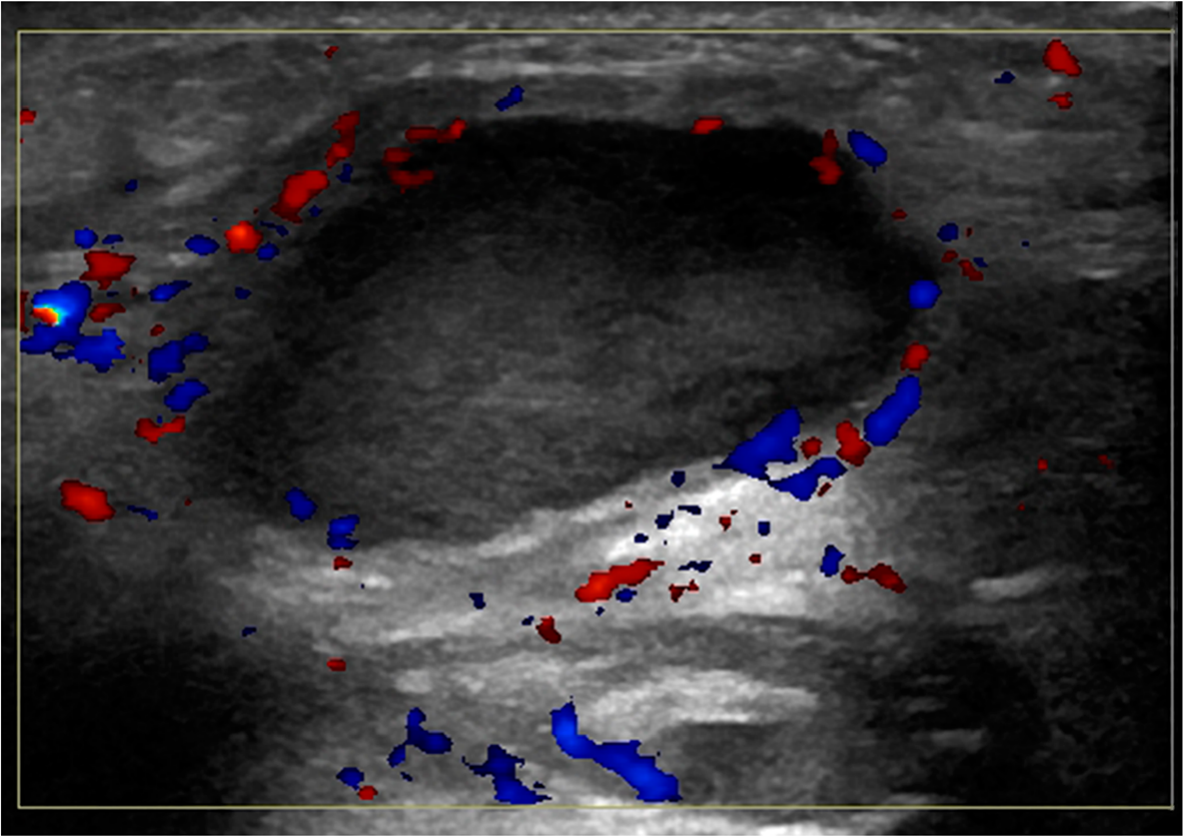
Infected thyroglossal duct cyst. A 23-year-old male presents with several days of fever, as well as a warm anterior neck mass. Long axis ultrasound image with color Doppler reveals a thick-walled hypoechoic structure containing low level echoes with peripheral hyperemia in the paramidline anterior neck
